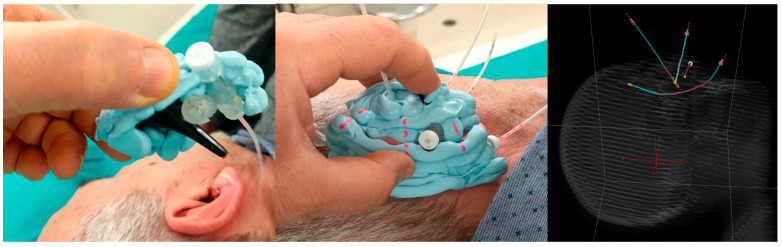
Three-dimensional CT reconstruction of the patient head and the Freiburg flap applicator adapted to the auricular region with hybrid implant configuration with the 4 catheters used (contact combined with intracavitary).
